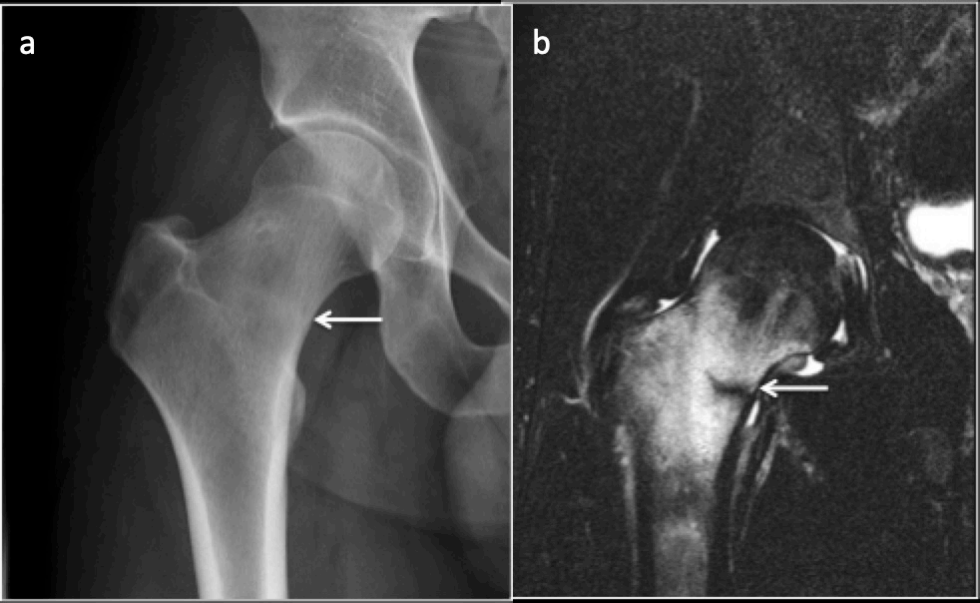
Fatigue fracture in the femoral neck where cancellous bone predominates. (a) Radiograph demonstrating subtle intramedullary sclerosis (arrow) along the fracture line with blurred trabeculae. (b) Coronal STIR image demonstrating marrow oedema and the linear hypointense fracture line(arrow). STIR, short tau inversion recovery.
